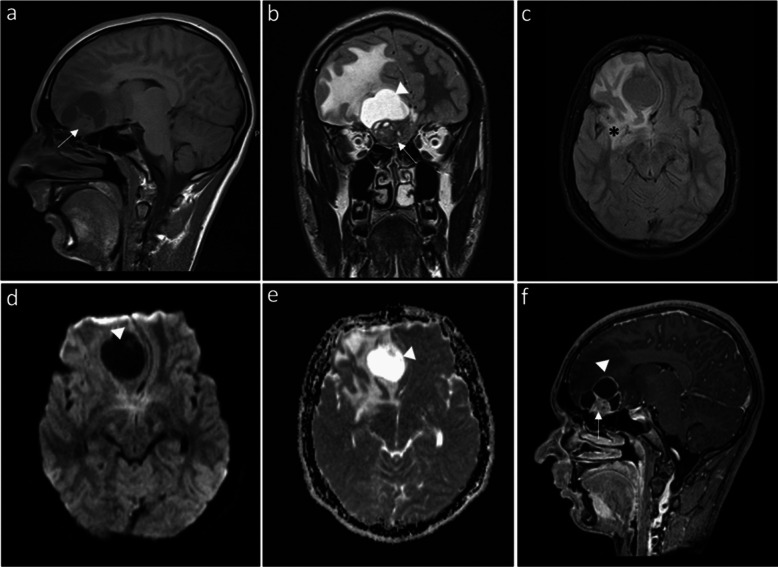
Brain magnetic resonance imaging in a 16-year-old boy shows a right extra-axial frontobasal tumor, attached to the cribriform plate, with a solid inferior component and a superior multiseptated cystic component. a Sagittal T1-weighted image shows the solid portion (*arrow*), isointense to the cortex. b Coronal T2-weighted image reveals a heterogeneously hypointense solid portion (*arrow*) with hyperintense microcystic foci and the superior cystic component (arrowhead). c T2-FLAIR image shows marked right frontal vasogenic edema (asterisk). d, e Axial diffusion-weighted image and corresponding apparent diffusion coefficient map demonstrate facilitated diffusion in the cystic component (arrowheads). f Sagittal post-contrast image shows marked enhancement of the solid portion (*arrow*) and peripheral linear enhancement of the cystic component (arrowhead)
